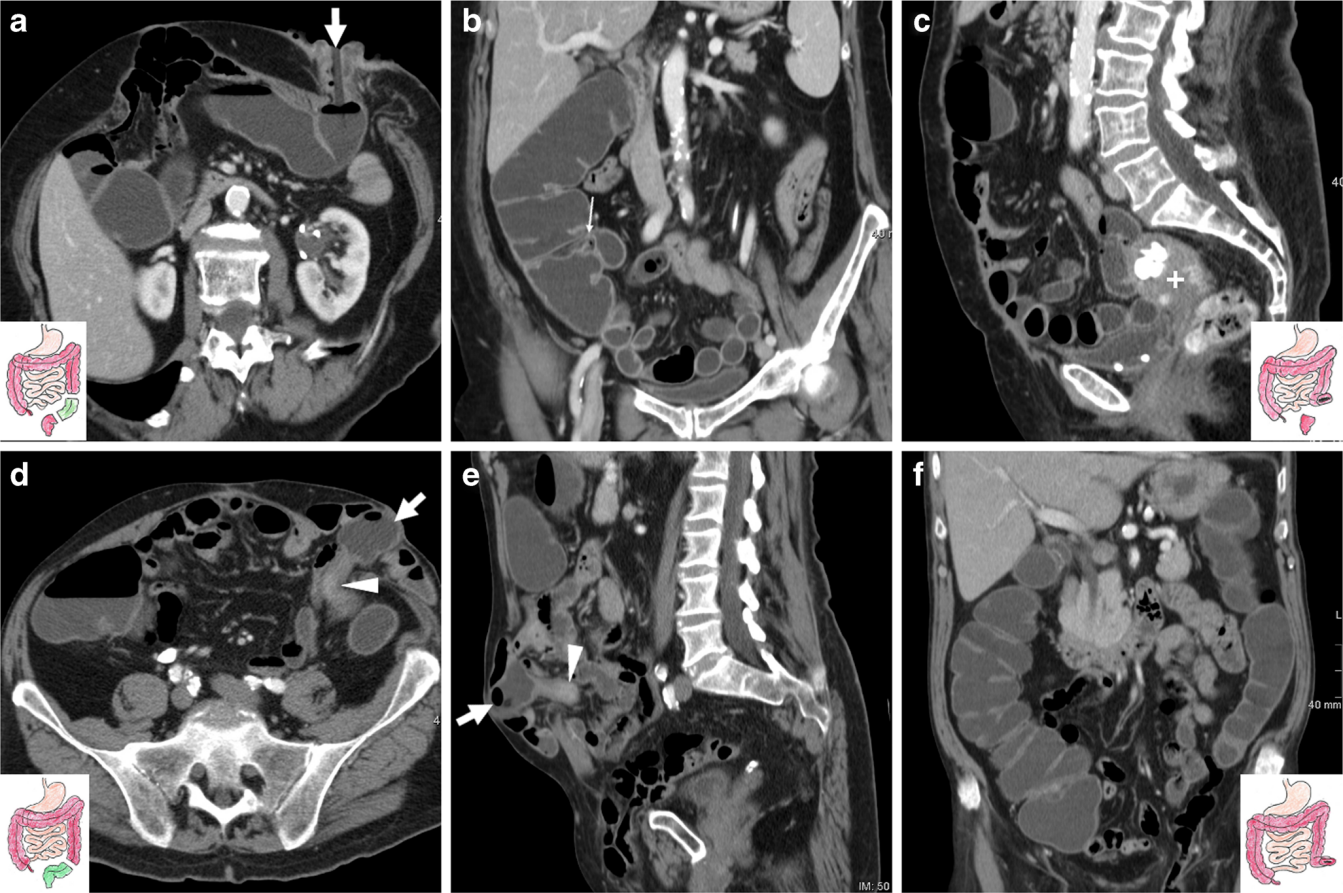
Two examples of water-enema multidetector CT (WE-MDCT) via the colostomy (surgical schemes shown in insets). a–c performed before elective bowel recanalisation in a 79-year-old female with previous Hartmann’s resection of the sigmoid and descending colon for complicated diverticulitis. Optimal retrograde distension of the residual large bowel up to the ileocecal valve (thin arrow in b) is achieved using a Foley catheter (thick arrow in a) positioned in the left-sided stoma. Note the closed rectal stump (+ in c). d–e) performed via the permanent colostomy in an 85-year-old male following abdomino-perineal resection for perforated rectal cancer (note absent rectum in e). Segmental poor distension of the pre-stomal tract (arrowheads in d, e) surrounded by stomal herniation of small bowel loops. Optimally distended descending, transverse and right colon (in f) without abnormalities
